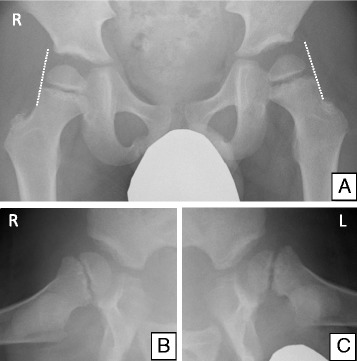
Radiological findings of the patient. Radiographs of the hip joint show Trethowan’s sign bilaterally in the frontal view (a) and a posterior tilting angle of 25° on the right side (b) and 34° on the left side (c) in the Lauenstein view
